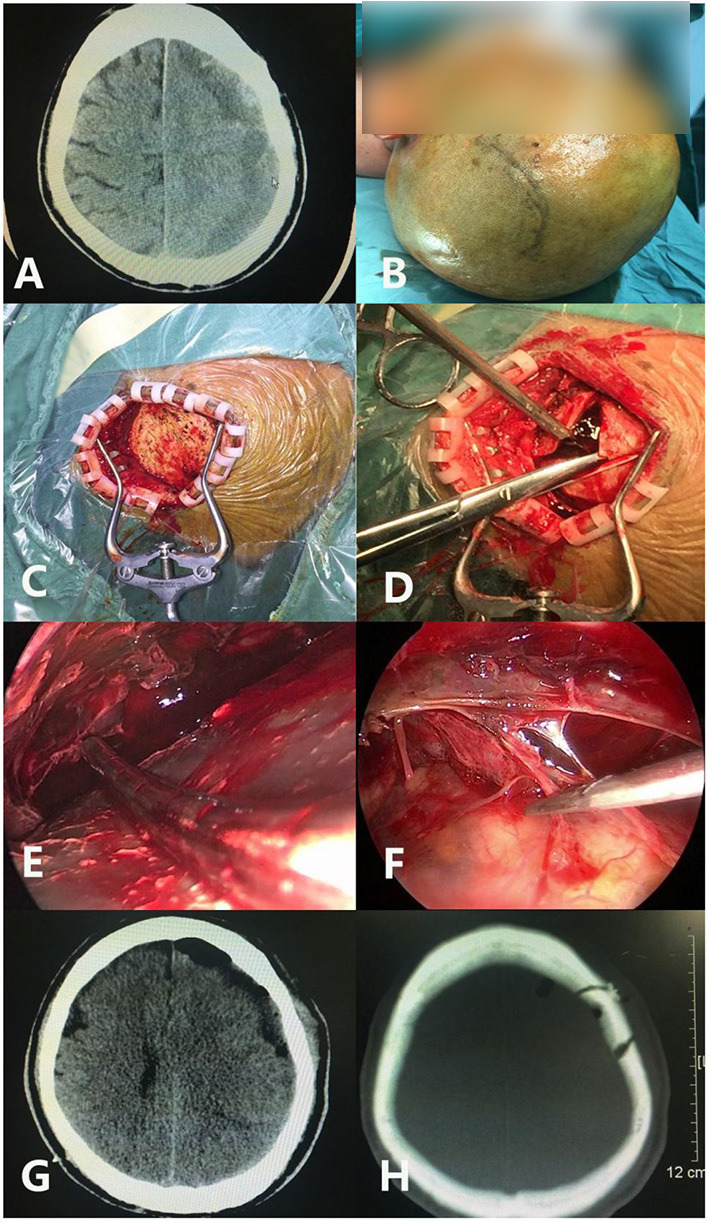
A case of septated chronic subdural hematoma under endoscopic evacuation. (A) Preoperative CT shows the subdural hematoma separation. (B) The skin incision is located at the coronal suture, with a backward ‘C’ shape. (C) The skin is open after incision. (D) After the valve is formed, the dura mater is cut in a cross shape. (E) The S shape of the suction device is used to push the brain tissue during the operation to expose the hematoma distant from the bone window. (F) The separation is cut. (G) Postoperative CT examination shows that the hematoma was removed satisfactorily. (H) The position, size (about 2–3 cm), and shape of the actual bone window is shown.
